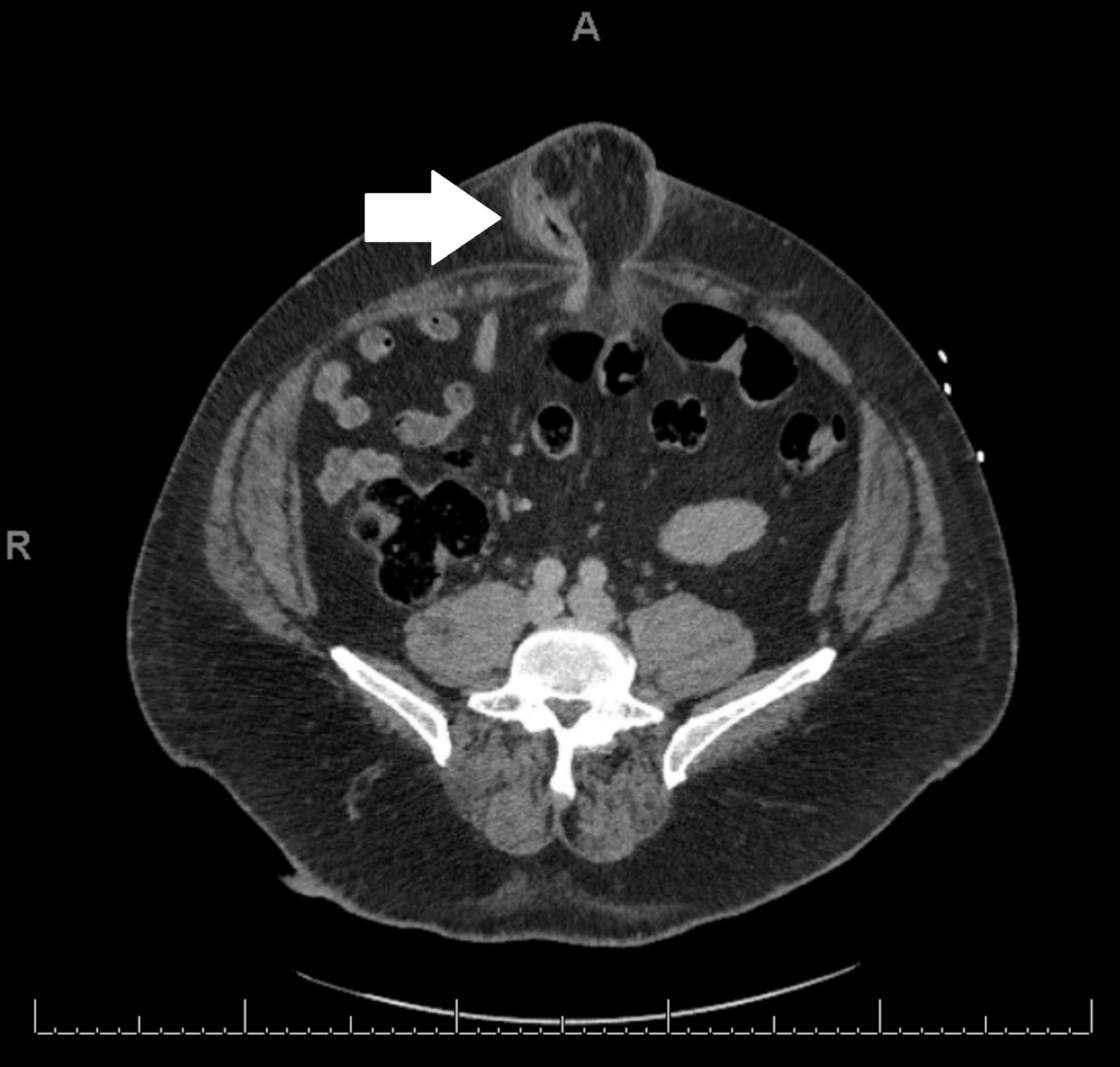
Axial CT showing umbilical hernia containing the appendix.
